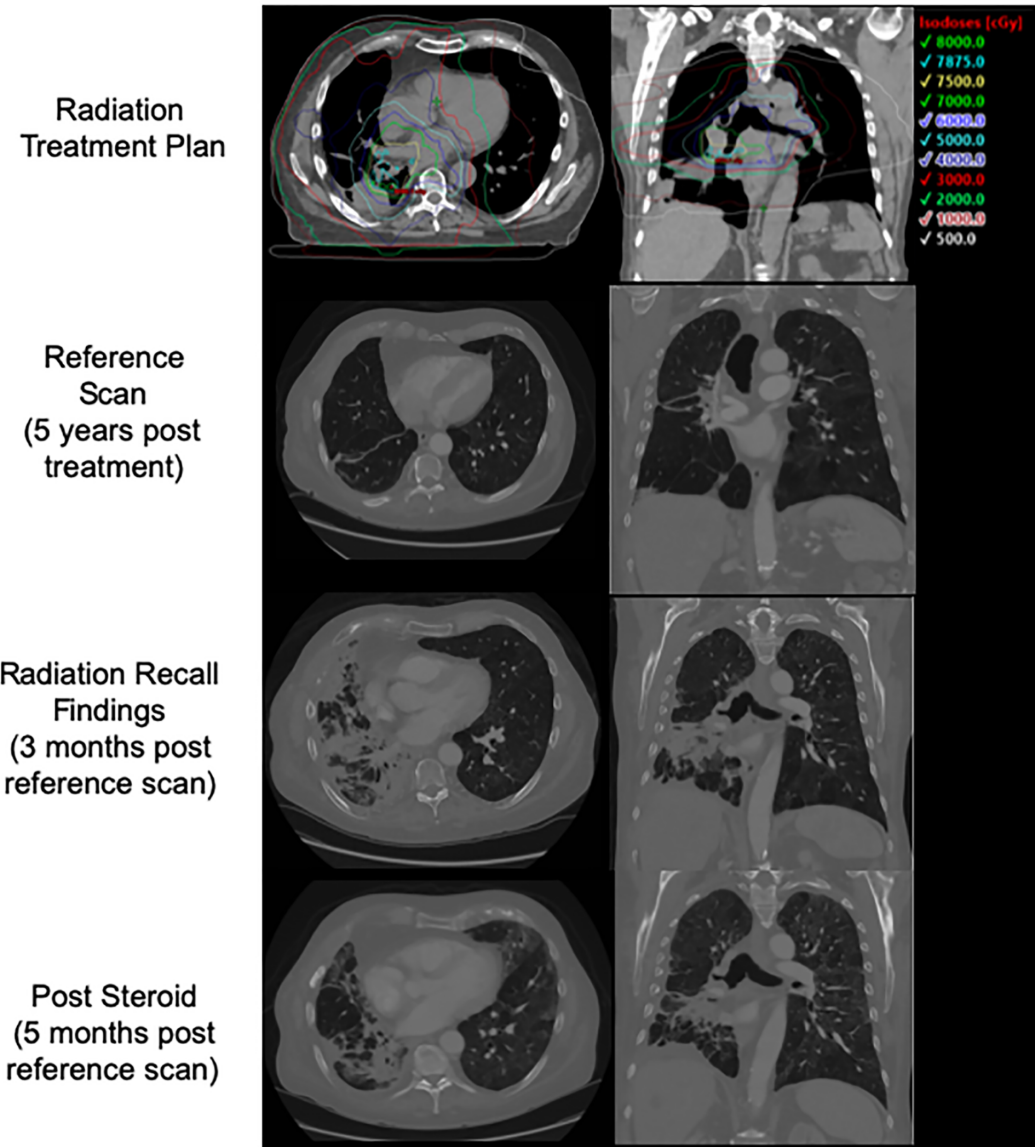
Patient 1's initial treatment plan, post treatment CT, CT at time of presentation with radiation recall, and CT following course of steroids with interval improvement.
